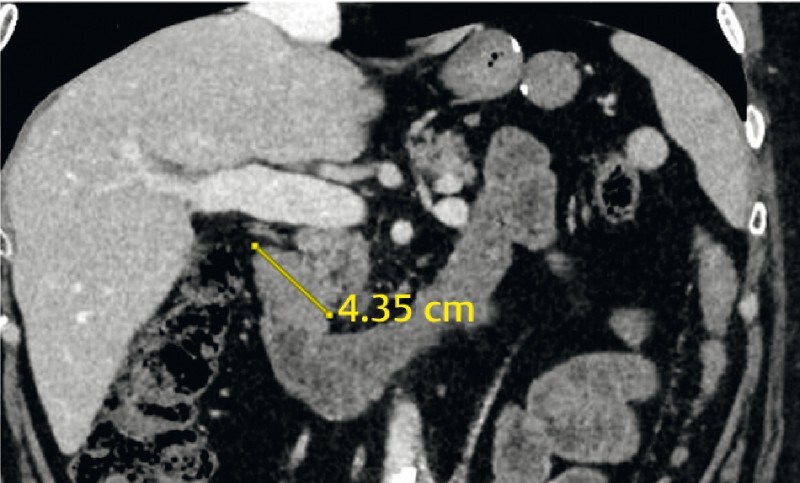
Computed tomography scan showing a 4.3-cm lesion in the first duodenum.
